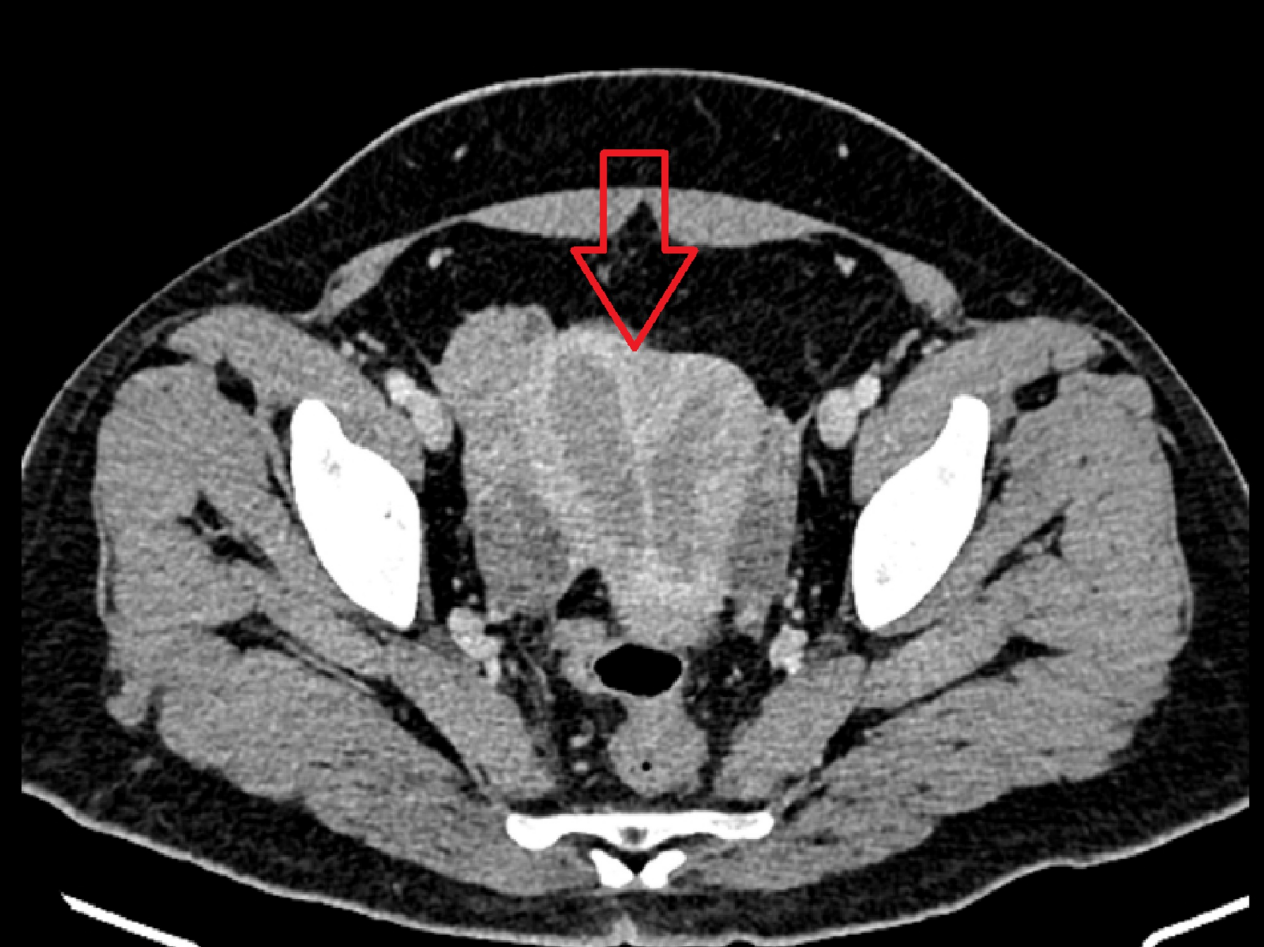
CT scan showing the presence of the tumor invading both uterine cavities with thickened walls (red arrow)
